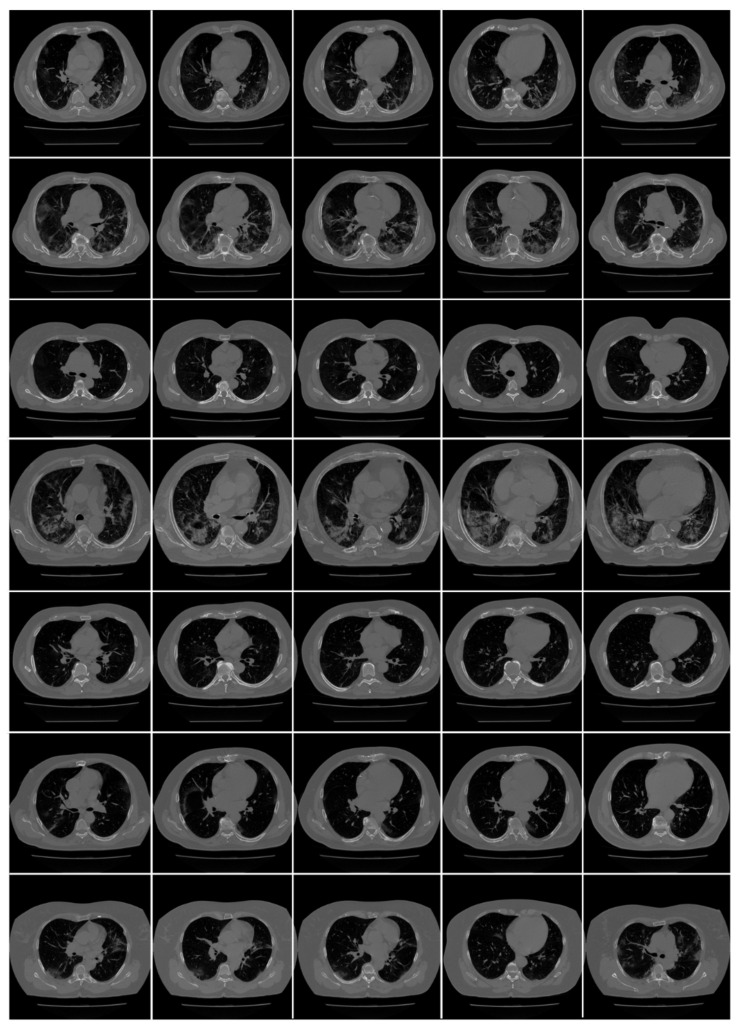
Raw CT images from the CROATIA dataset.
